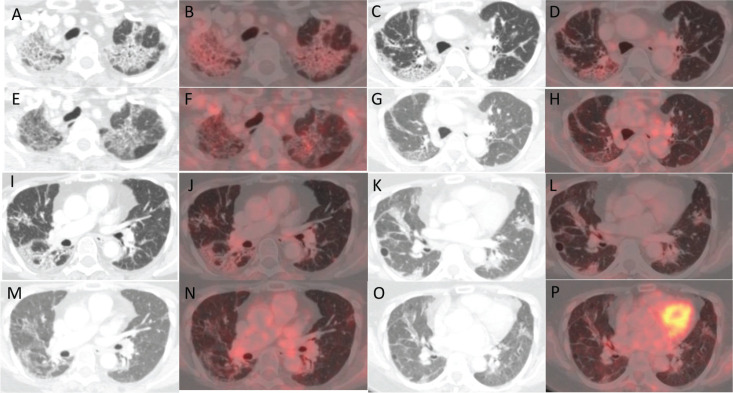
(a, c, i and k): Axial CT images of four different sections of lung showing changes with increased metabolic activity in corresponding (b, d, j and l): axial fused PET-CT images in the PET-CT scan after treatment with pembrolizumab. (e, g, m and O): Axial CT images of the same four sections of lung showing a reduction in the previously noted changes with reduced metabolic activity in corresponding (f, h, n and p): axial fused PET-CT images in the PET-CT scan done post treatment with pembrolizumab and tocilizumab.
